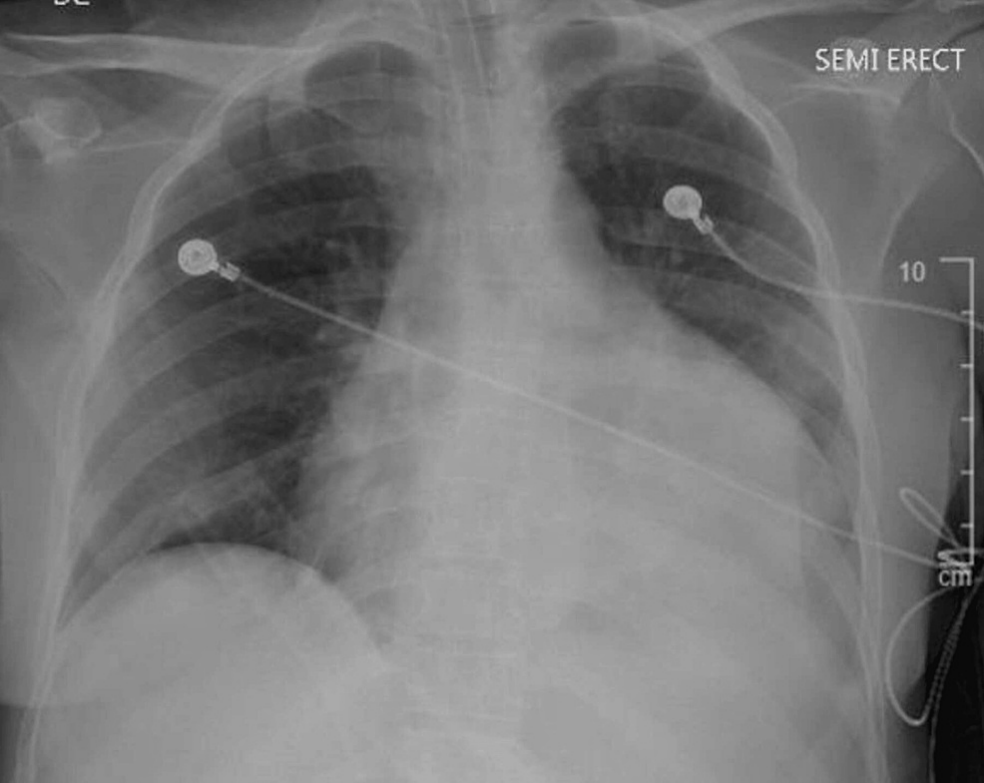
Chest X-ray on admission showing left perihilar and lower lobe infiltrates concerning pneumonia and cardiomegaly.
